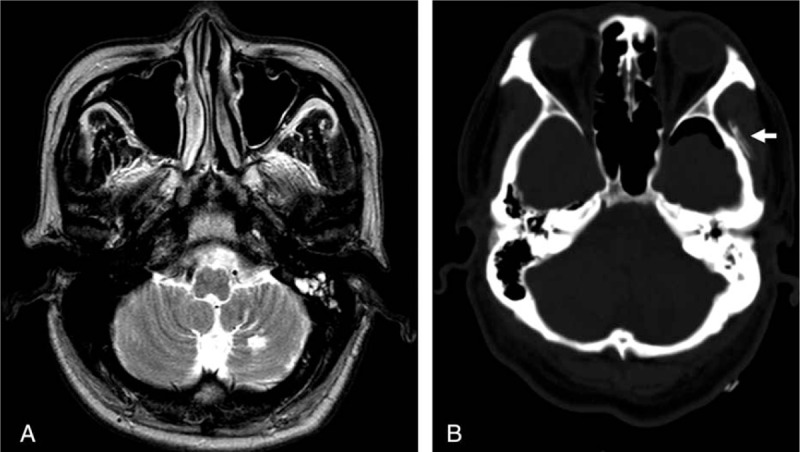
Preoperative axial T2-weighted magnetic resonance imaging shows a focal encephalomalatic change in the left cerebellar hemisphere due to previous ischemic stroke (A). Bone setting of the immediately postoperative head CT shows that 1 drain string was placed just above the temporal bone-work side (arrow) (B). CT = computed tomography.
